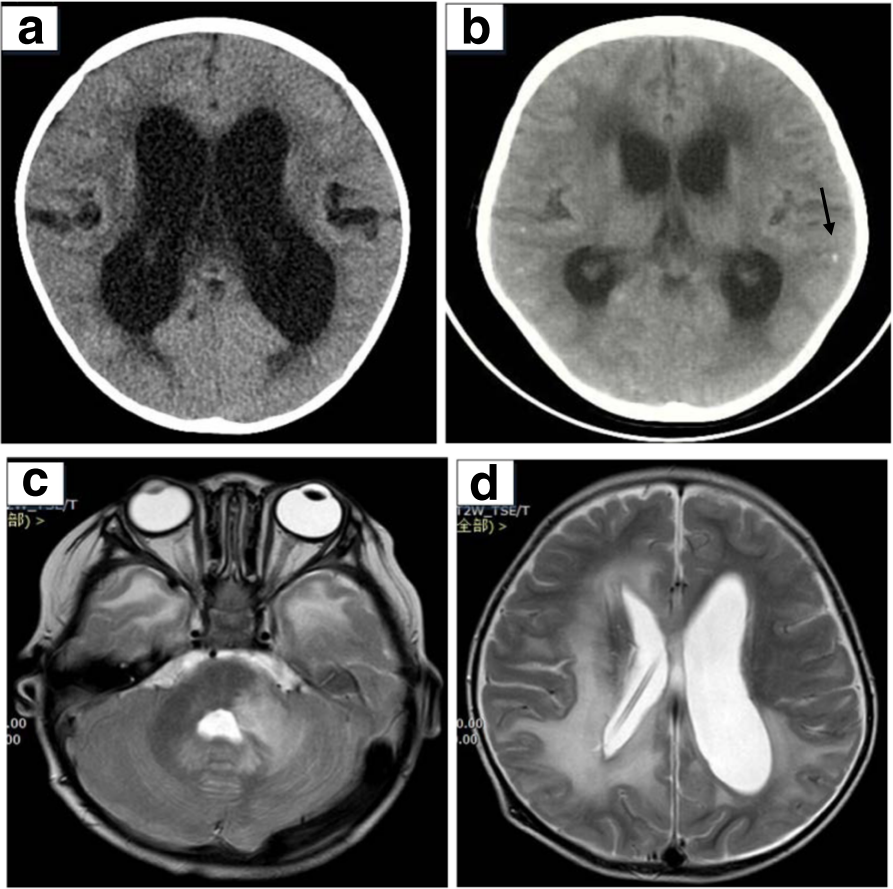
Cranial CT scan and MRI. a Hydrocephaly. b Calcification spots in brain parenchyma. c Abnormal signals in cerebral parenchyma. d Improved hydrocephaly after treated by lateral external ventricular drain
